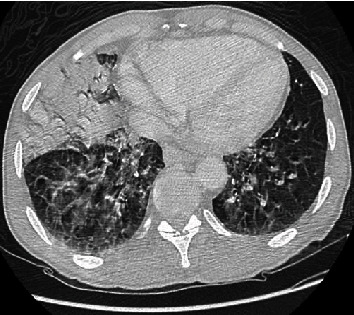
CT chest showing bilateral ground-glass opacities and right middle lobe consolidation.
